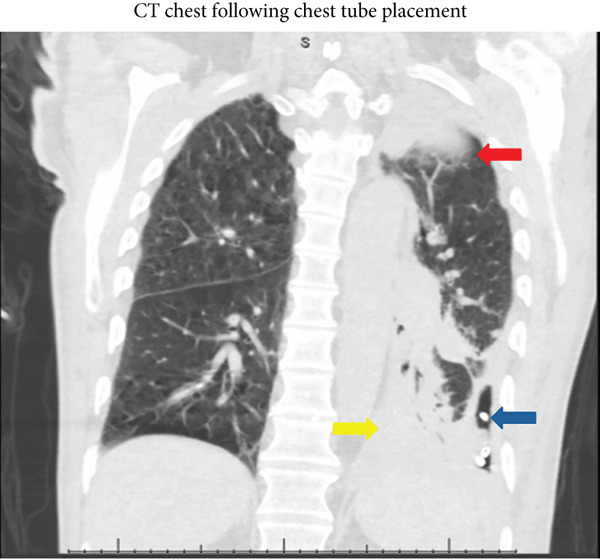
A CT chest in the coronal view after chest tube placement and drainage displaying a reduced basilar collection (yellow arrow) with pigtail catheter in place (blue arrow) and a persistent apical collection (red arrow).
